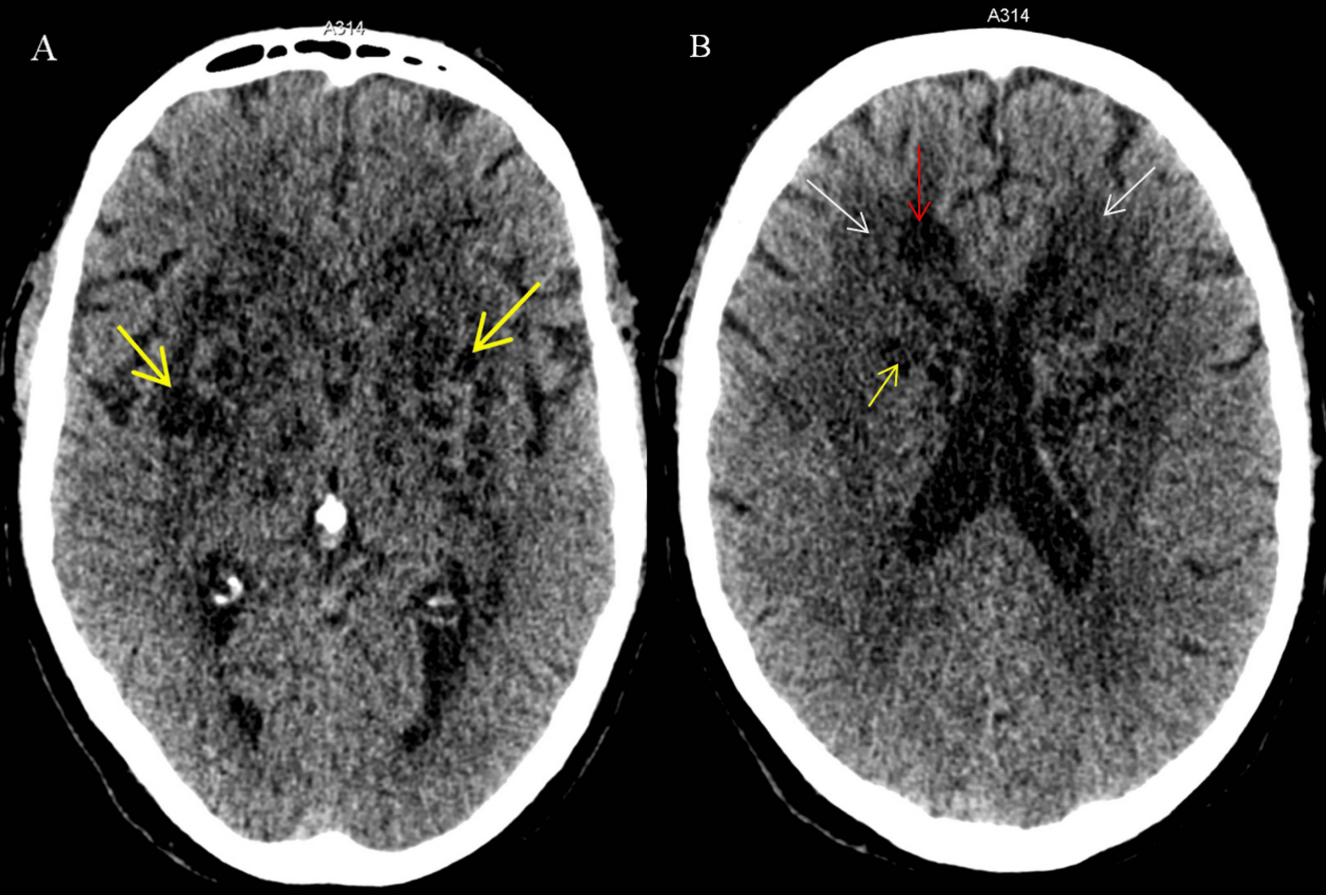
Axial brain CT scan without contrast injection (A) A bubbly appearance due to multiple bilateral cystic lesions in the basal ganglia, predominantly affecting the lenticulostriate region (yellow arrows). (B) Increased hypodensity of the periventricular white matter (white arrow), along with a unilateral hypodense lesion near the right frontal horn of the lateral ventricle in the right frontal lobe (red arrow), in association with multiple cystic lesions within the lenticulostriate region (yellow arrow).
